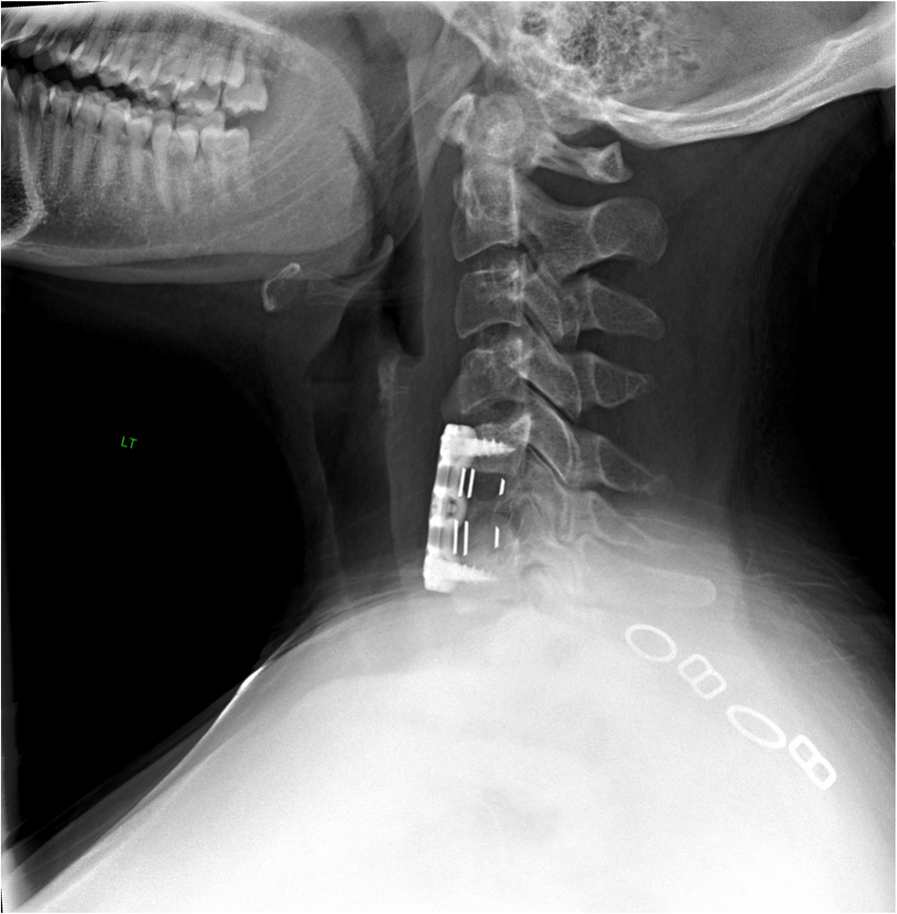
Postoperative lateral cervical spine X-ray showing 18 mm polyetheretherketone cage and 35 mm anterior cervical plate
